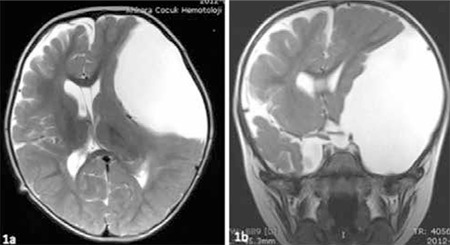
a,b) Axial/ Coronal MRI scan of the patient I showing a large middle cranial fossa arachnoid cyst, its stalk is deviated to the right side with midline shift (T2 weighted image)
